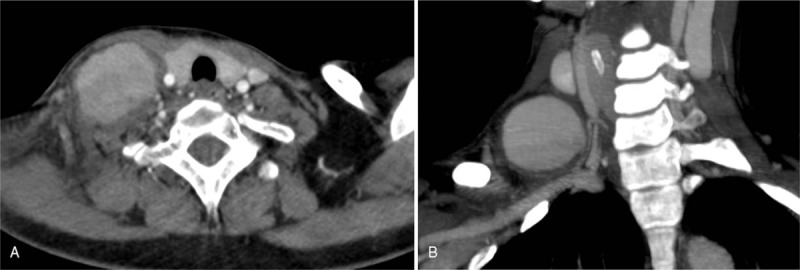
Cervical computed tomography angiography in axial (A) and sagittal (B) view, showing a large false aneurysm of the neck, with a likely origin from the thyrocervical trunk, with no signs of peri-arterial bleeding or hematoma.
